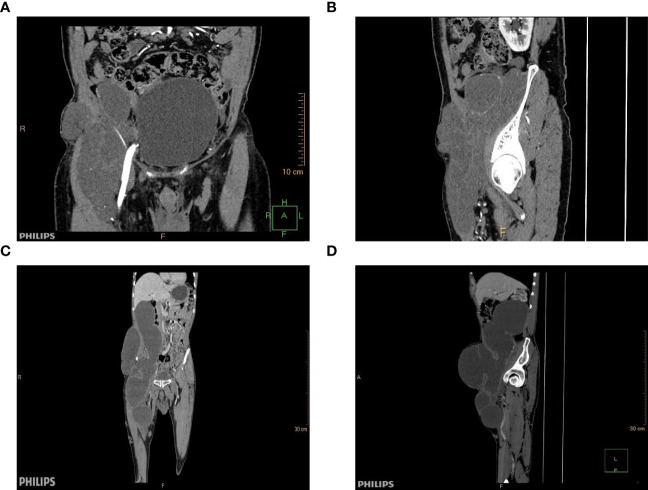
Contrast-enhanced CT image before and after three years. (A) Coronal contrast-enhanced CT image obtained in 2020. (B) 2020 contrast-enhanced CT image of a dislocation. (C) Coronal contrast-enhanced CT image obtained in 2023. (D) Contrast-enhanced CT image of a dislocation.
